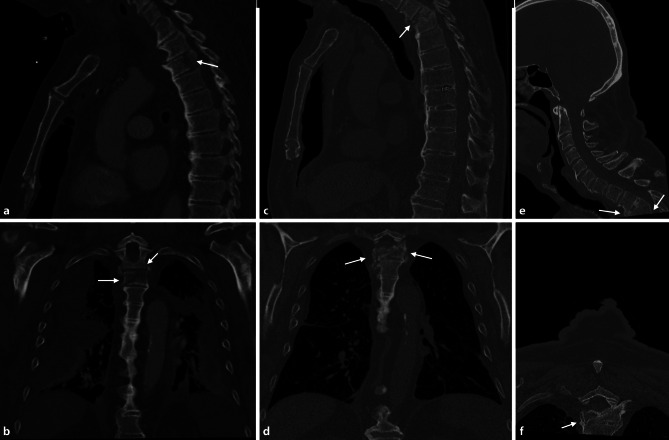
Follow-up images showing no apparent fracture healing at 18 days following trauma (thoracic CT scan; a sagittal plane,  b frontal plane), progressive destruction of the T3 vertebra at 3 months (thoracic CT scan; c sagittal plane, d frontal plane), and finally intersegmental fusion after 41 months (cranial CT scan down to cervicothoracic junction; e sagittal plane, f axial plane). *Arrows* mark T3 vertebra
